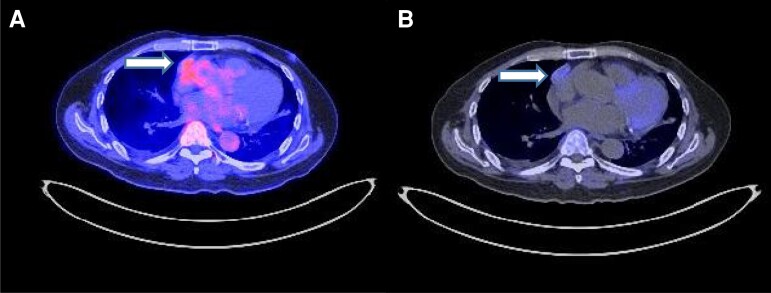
^18^FDG & ^68^Ga-DOTATATE positron emission tomography (PET)/CT at presentation demonstrating a right anterior mediastinal mass of 3.3 cm × 1.2 cm, with mild dual-tracer avidity. A, image with ^18^FDG tracer. B, image with ^68^Ga-DOTATATE tracer.
